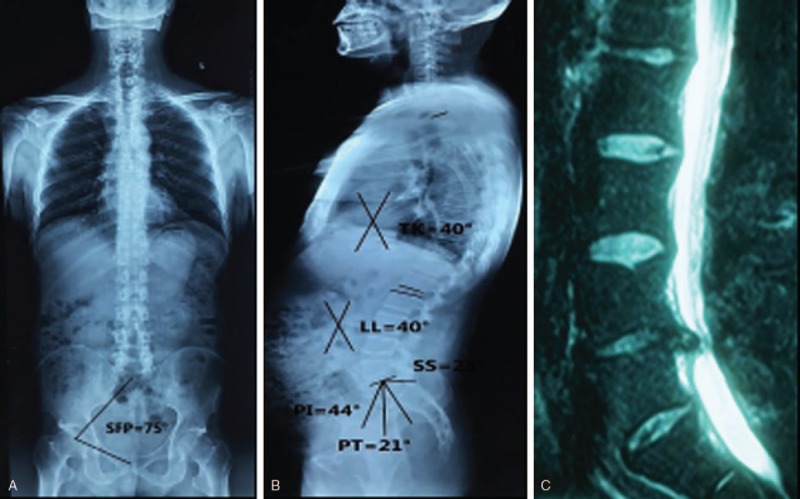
A male, 47 years old. SFP = 75°, PI = 44°, PT = 21°, SS = 23°, LL = 40°, TK = 40°. (A) Positive full spine X-ray; (B) lateral full spine X-ray; (C) lateral lumbar MRI showed disc herniation at L4/L5 level. LL = lumbar lordosis, PI = pelvic incidence, PT = pelvic tilt, SFP = sacrum-femoral-pubic symphysis, SS = sacral slope, TK = thoracic kyphosis.
